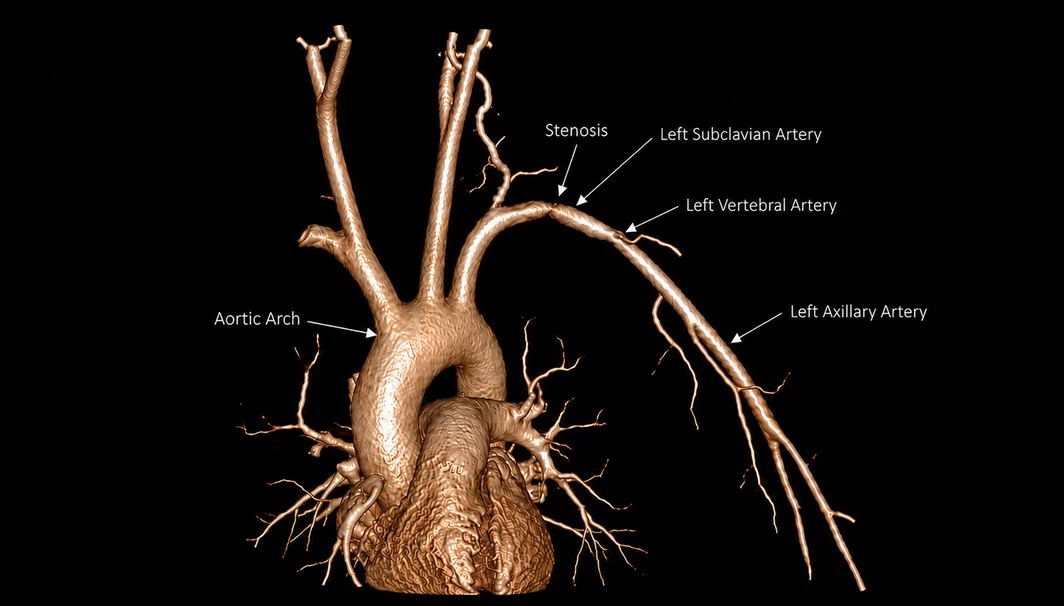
(3D‐CT Angiogram) Thrombosed graft with stenosis in the distal left subclavian artery.
